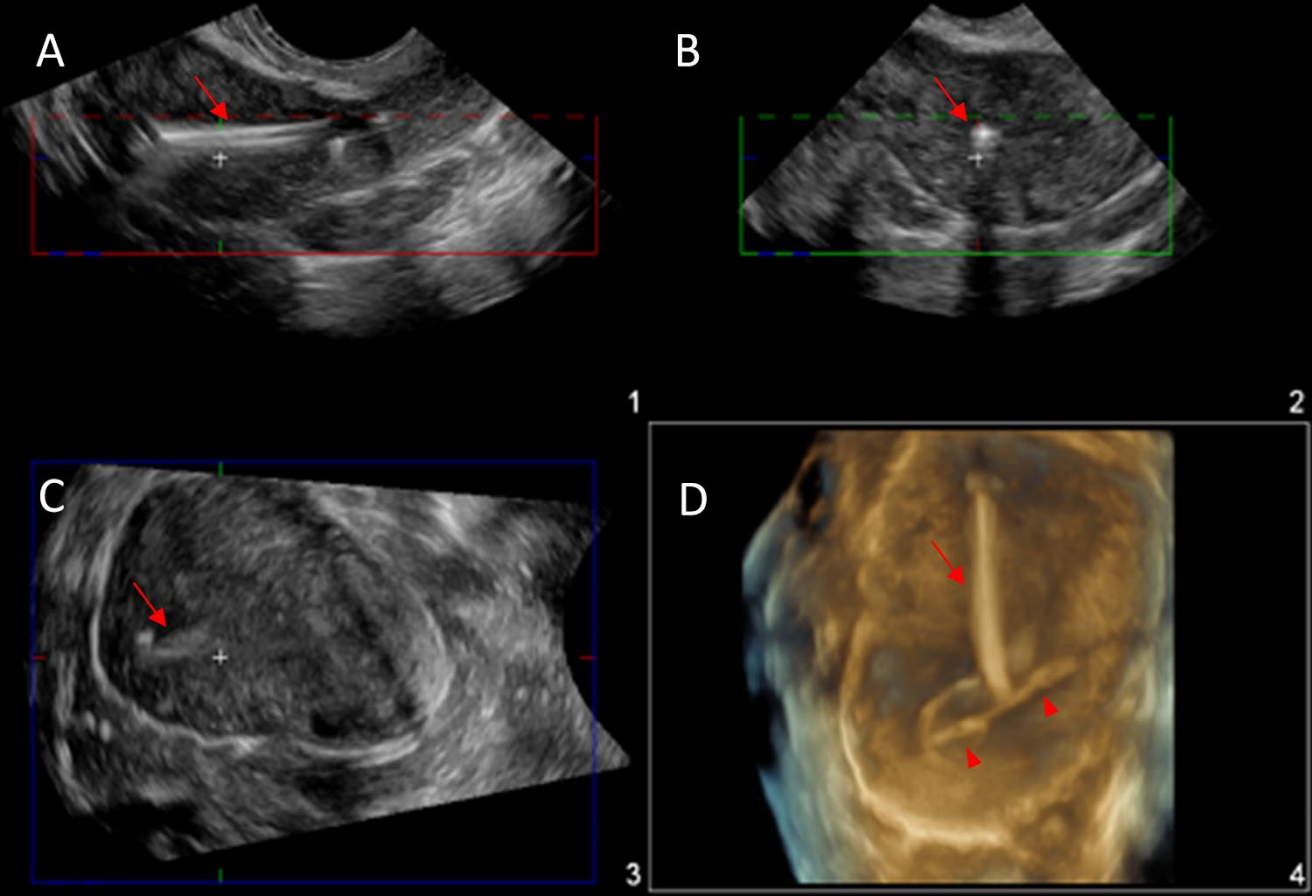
Malpositioned IUD. 2D grayscale transvaginal ultrasound images of the uterus in long (A) and short axis (B) demonstrating an IUD stem (arrow) centered within the endometrial cavity but arms not at the uterine fundus. 3D grayscale (C) and gynecologic tissue rendered (D) ultrasound images of the same patient demonstrating a flipped device with the IUD arms (arrowheads) extending outward within the lower uterus near the internal cervical os
